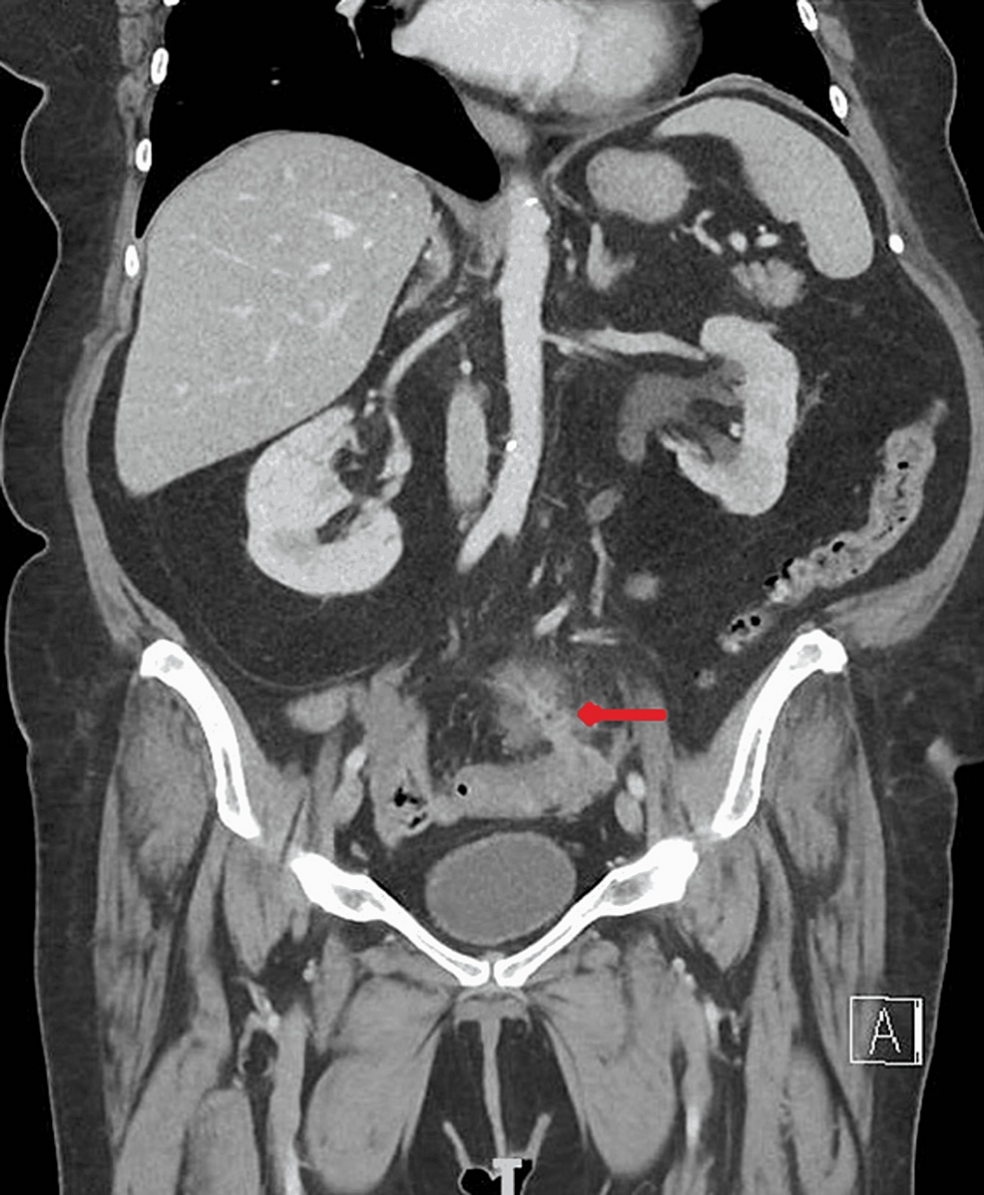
CT of the abdomen and pelvis showing focally perforated diverticulitis of the sigmoid colon (red arrow)
